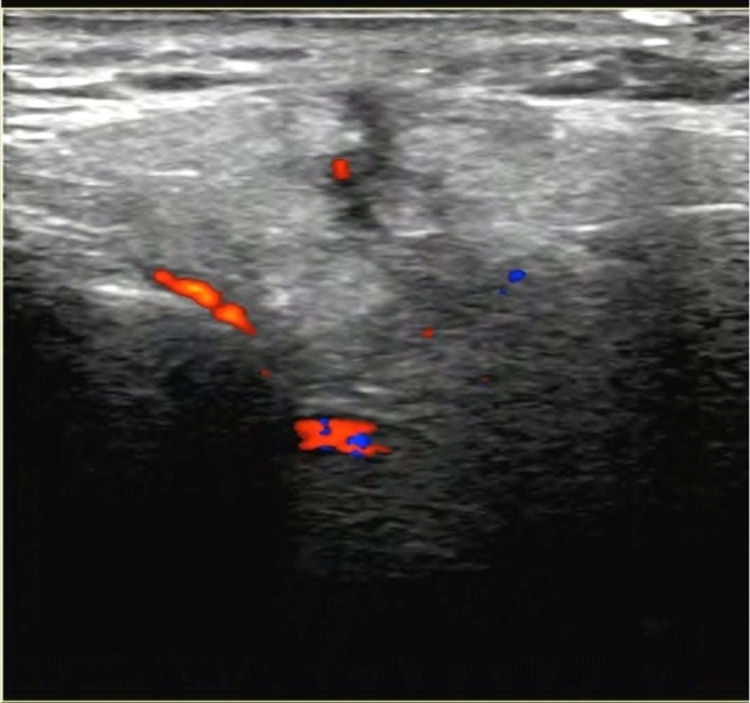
Ultrasound scan of the parotid gland shows a linear hypoechoic focus in the superficial parotid gland extending to the subcutaneous tissue
